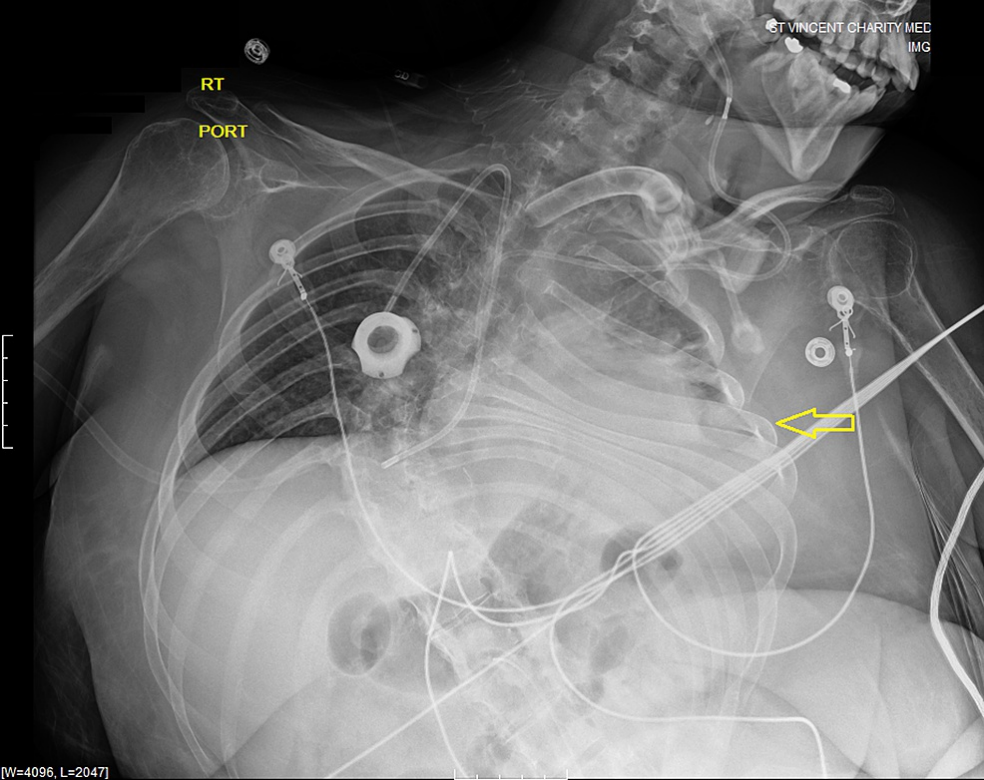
Chest X-ray. A single anteroposterior portable radiograph of the chest was obtained on admission. It showed a right internal jugular CVC with the tip overlying the cavoatrial junction, evidence of a tracheostomy tube present in situ, and a shunt catheter over the chest. Severe S-shaped scoliosis of the thoracolumbar spine and dense airspace consolidation over the left lung base was noted. CVC: central venous catheter
